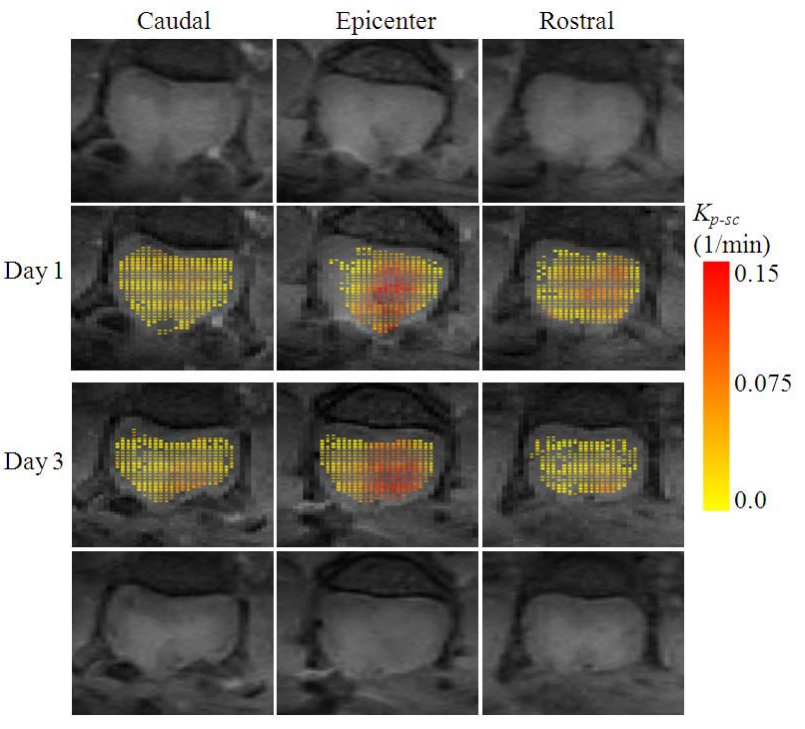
Color coded *K*_*p*-*sc*_-maps computed using Eq. 4 from the three slices centered at the epicenter using the data set in Figure 4. The backgrounds are the T1-weighted precontrast images (top and bottom rows). The color from yellow to red indicates linearly increasing compromise in blood spinal cord barrier permeability. The areas with color towards red indicate regions with more vascular damage causing the barriers to become more permeable to blood constituents.
